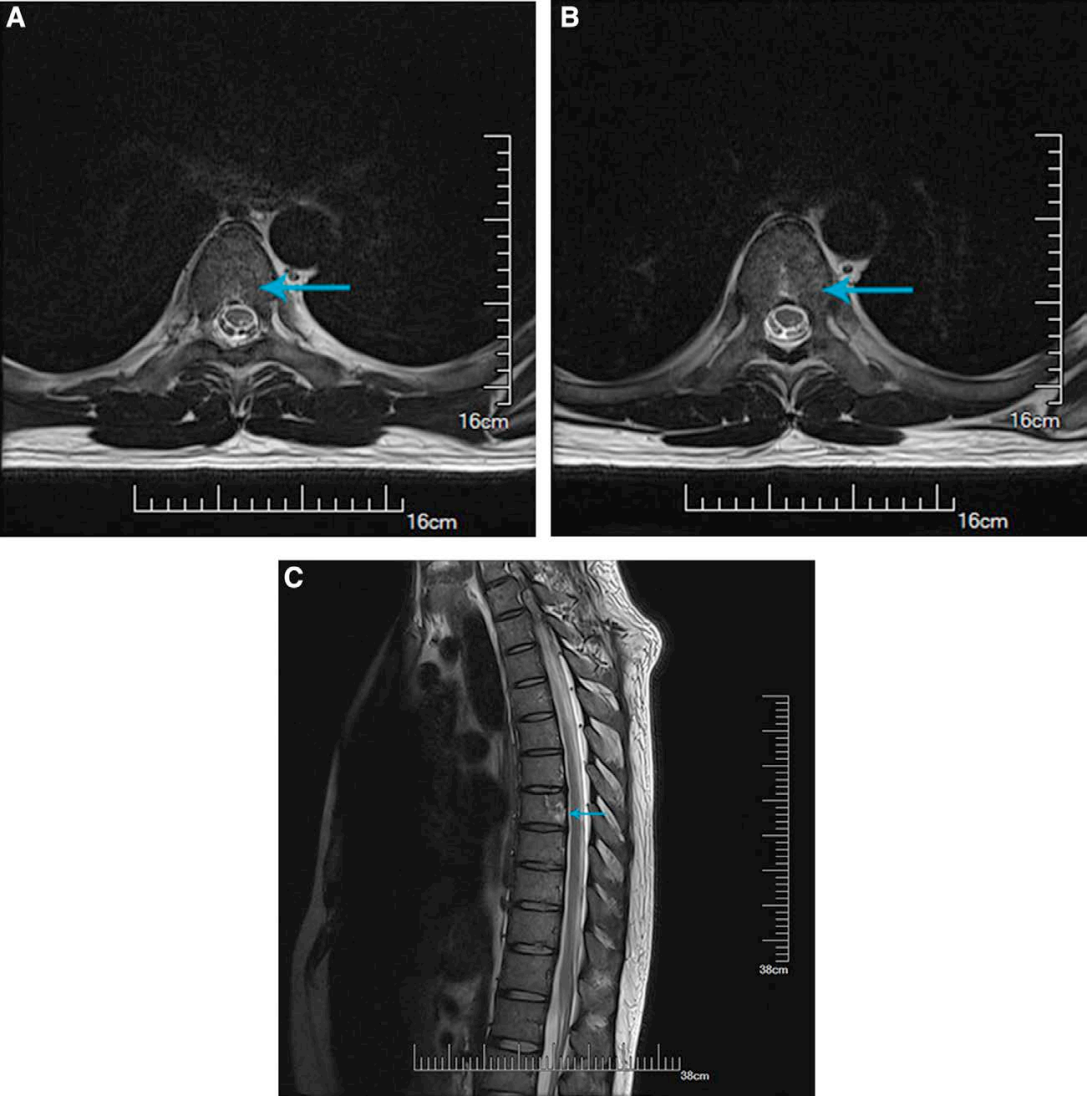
Axial MRI T2WI sequence showing improvement of the high signal lesion in spinal cord and vertebrates after treatment. MRI = magnetic resonance imaging, T2WI = T-2 weighted imaging.
